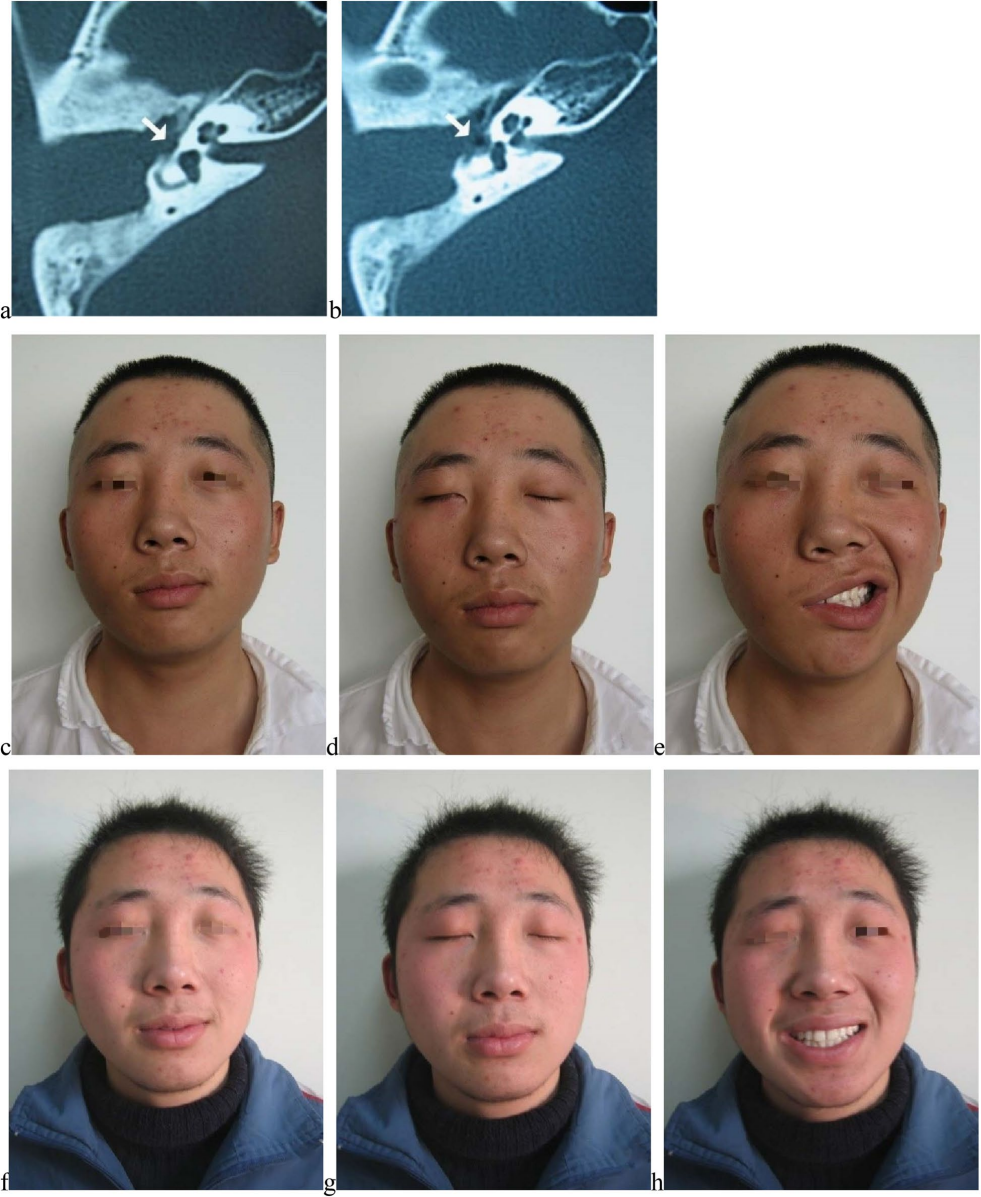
Recovery from H-B grade V to H-B grade I after decompression. No. 12 patient, male, 17-year-old, suffered from facial nerve edematous in the canal wall down mastoidectomy for right-side cholesteatoma of middle ear, received decompression within 2 months. a b Axial CT images revealed fallopian canal of tympanic segment was defective. c d e Facial images before decompression; c at rest before decompression; d eye closure before decompression; e smile before decompression. f g h Facial images after decompression; f at rest after decompression; g eye closure after decompression; h smile after decompression
